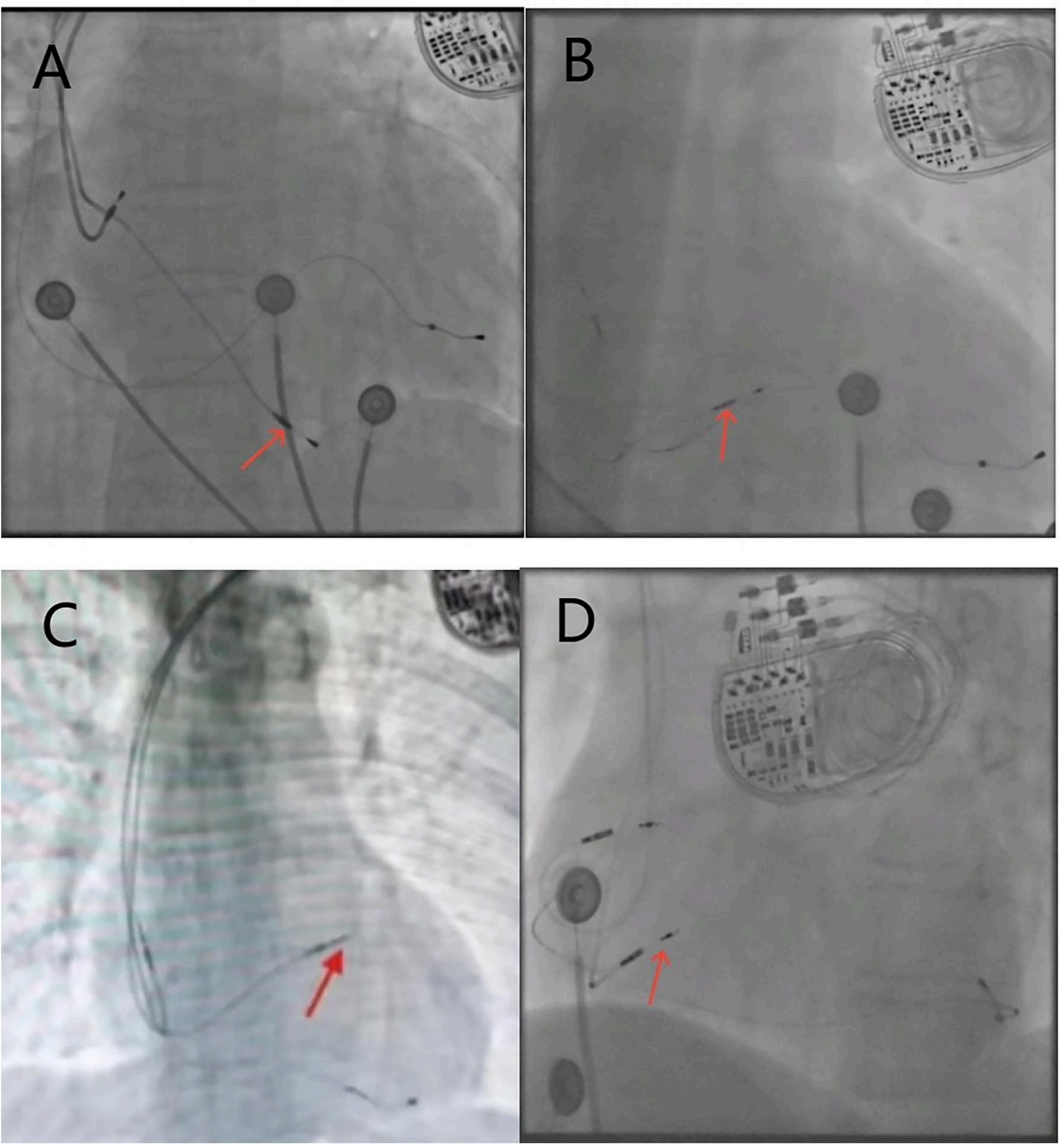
X-ray fluoroscopy images of the right ventricular electrode position, with red arrows indicating the implantation site of the RV electrode. (A) Posteroanterior view of the low-septum; (B) posteroanterior view of the mid-septum; (C) posteroanterior view of the high-septum; (D) left anterior oblique view of the left bundle branch area.
